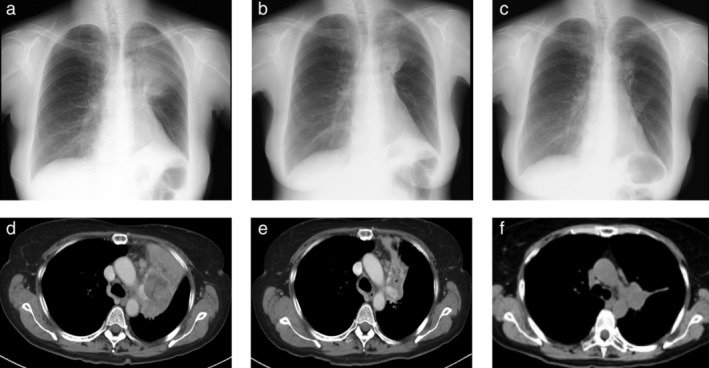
(a–c) Chest roentgenography and (d–f) computed tomography. (a,d) The primary tumor was observed in the left upper lobe before the initiation of pembrolizumab treatment. (b,e) At the onset of fulminant type 1 diabetes mellitus (T1DM) after the eighth course, the mass had reduced. (c,f) Continued pembrolizumab treatment further reduced the size of the primary lesion after the 18th course.
